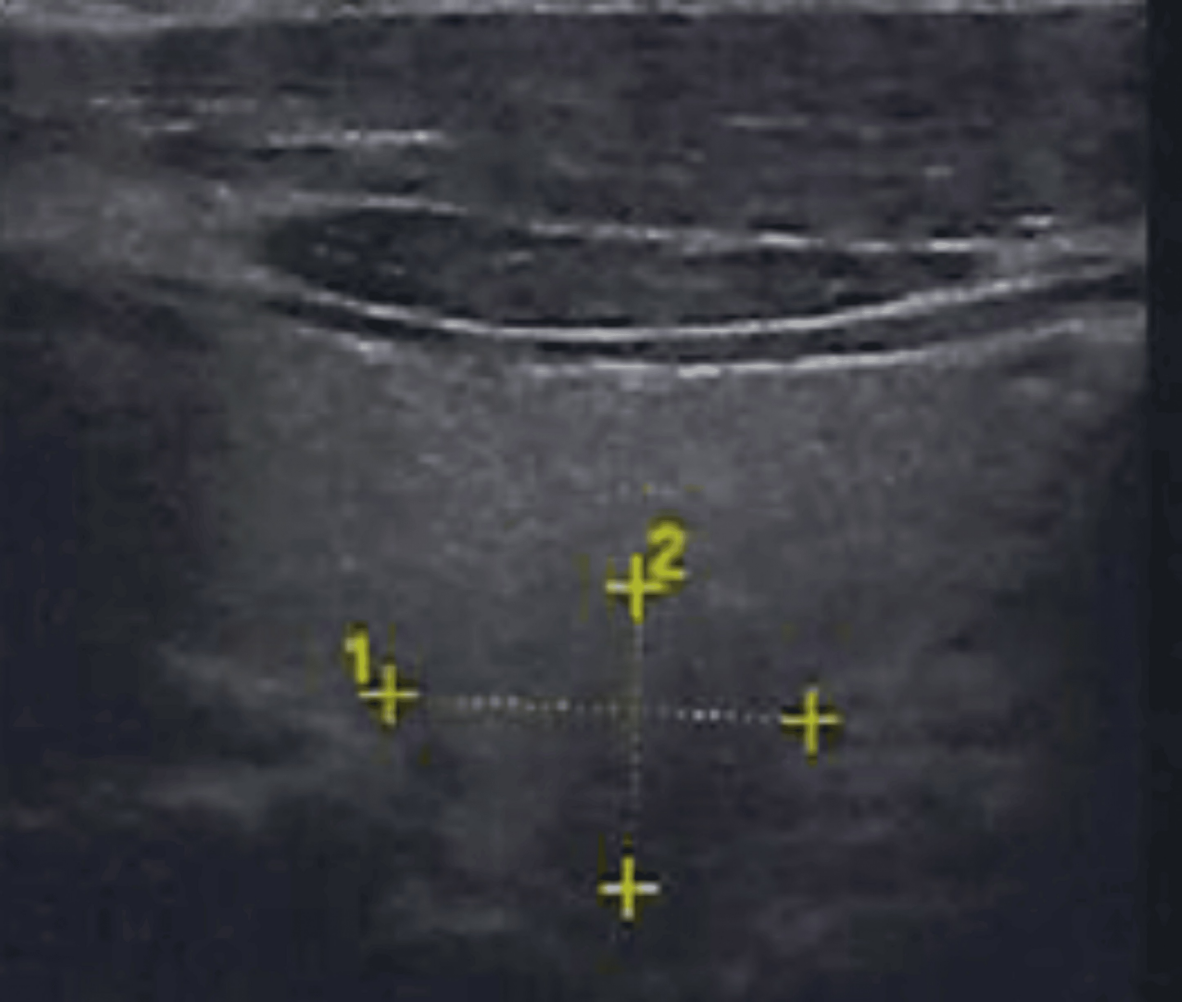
Neck ultrasound showing a solid nodular area in the right thyroid lobe; fine-needle aspiration (FNAB) classified it as Bethesda System Category II. The Bethesda System for Reporting Thyroid Cytopathology [4]
